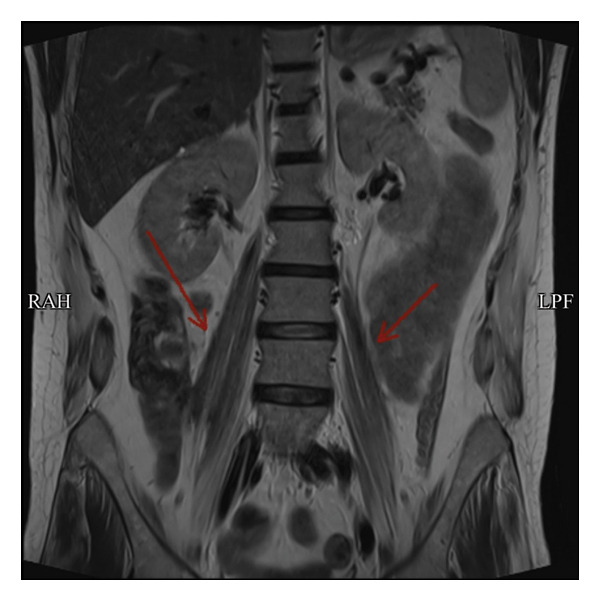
Coronal T2‐weighted MRI image showing diffuse atrophy and fatty replacement of the psoas muscles (indicated by the red arrow).
